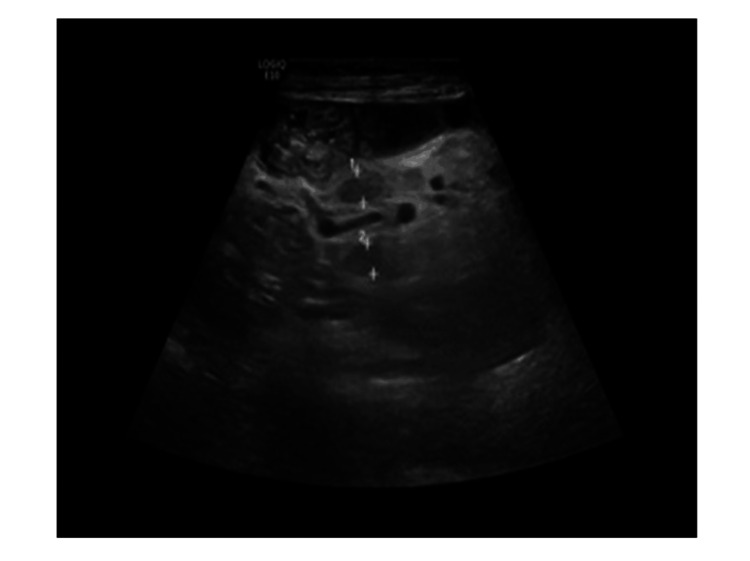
Ultrasound image showing an expanded jejunum with hyperechoic mesentery and multiple reactive lymph nodes.
